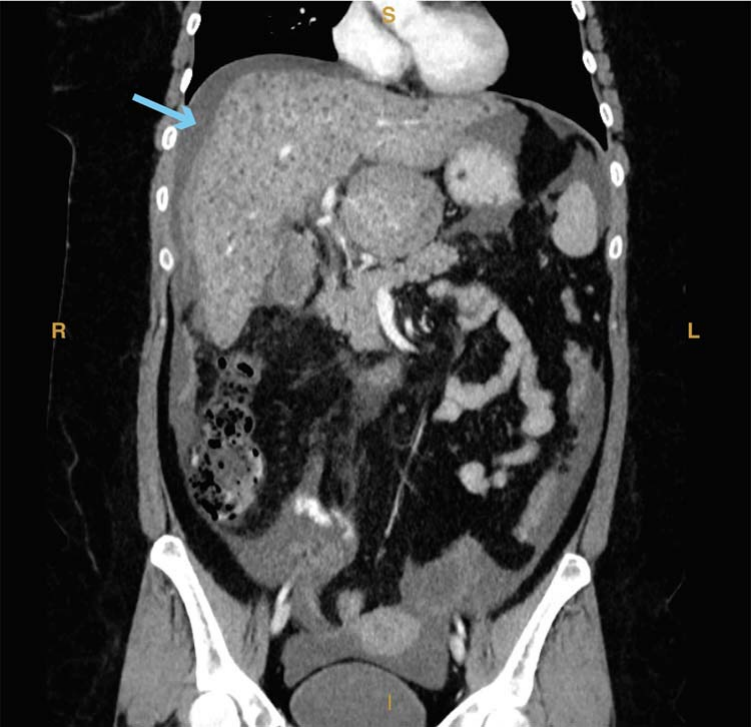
Computed tomography imaging from Case 3 showing significant hemoperitoneum (cyan arrow). Present in the figure, the letters S, L, I and R denote superior, left, inferior and right respectively.
